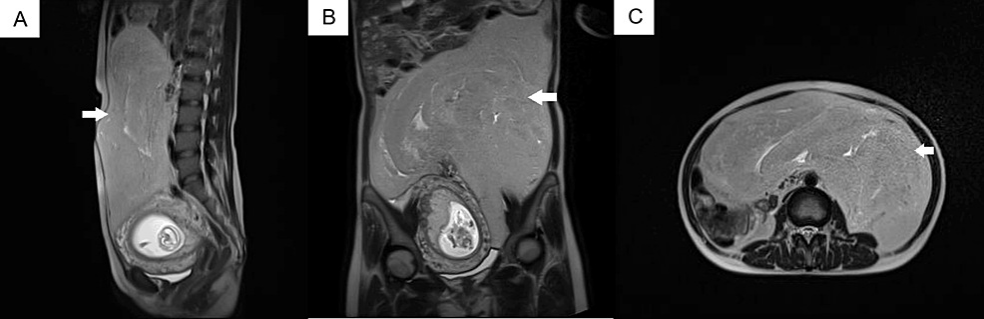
Axial (A), coronal (B), and sagittal (C) magnetic resonance imaging (MRI) images of massive retroperitoneal liposarcoma
